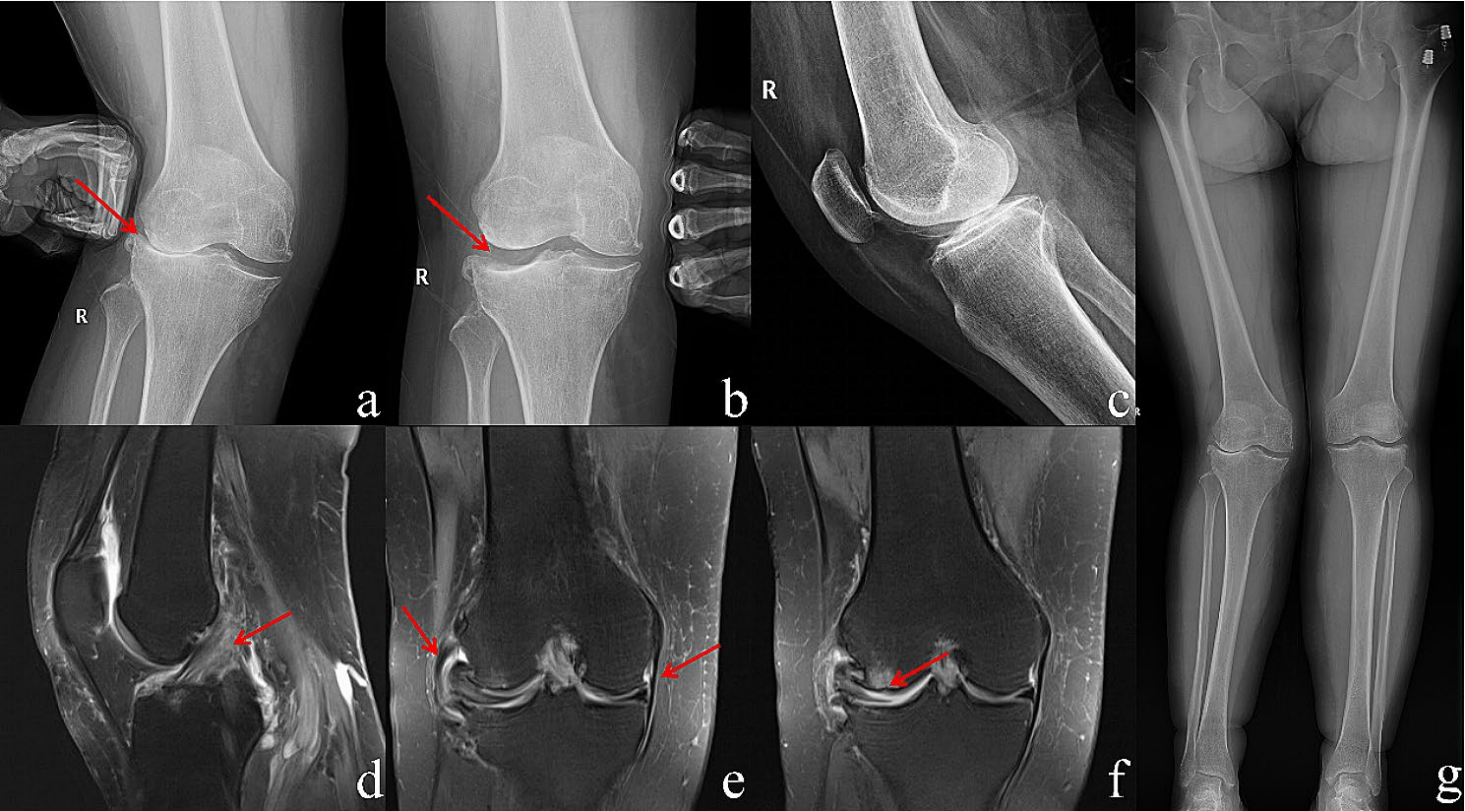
Preoperative imaging examination for LUKA Preoperative X-ray and knee MRI examinations are necessary to assess the suitability of the surgery. X-ray can initially identify simple lateral compartment osteoarthritis (a, b, c, and g) and determine if valgus correction is possible (b). MRI can confirm the condition of the ACL (d) and medial and lateral collateral ligaments (e) and verify the presence of worn cartilage only in the lateral compartment (f)
